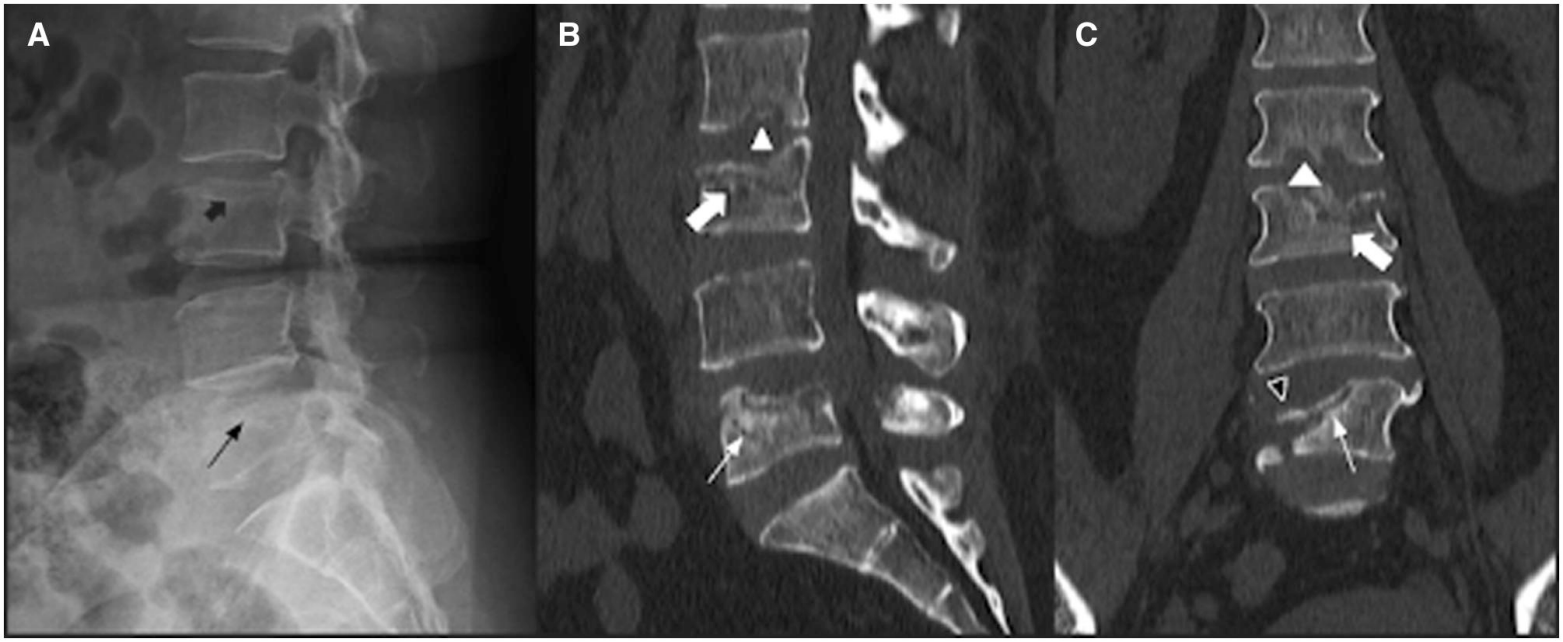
Plain radiograph and CT scan images of lumbosacral spine show subchondral endplate fractures with collapse of vertebral bodies—subchondral fracture of the superior endplate and reduced height of the L3 vertebra (black thick arrow) and irregular superior endplate with collapse of body and reduced height of the L5 vertebra (black thin arrow) is seen on a lateral radiograph (A), Subchondral fracture of inferior endplate of L2 (white arrowhead), subchondral fractures of superior endplate of L3 and L5 vertebra with reduction in height (white thick and thin arrows) and collapse of the right lateral aspect of the L5 vertebral body (black arrowhead) seen on sagittal and coronal plane images of CT scan (B) and (C).
